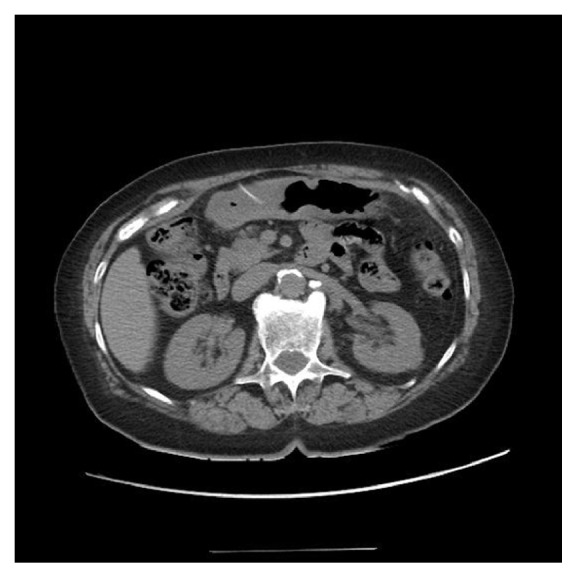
CT image of abdomen and pelvis without contrast, revealing a hyperdense, elongated, linear foreign body perforating the serosa of the stomach.
